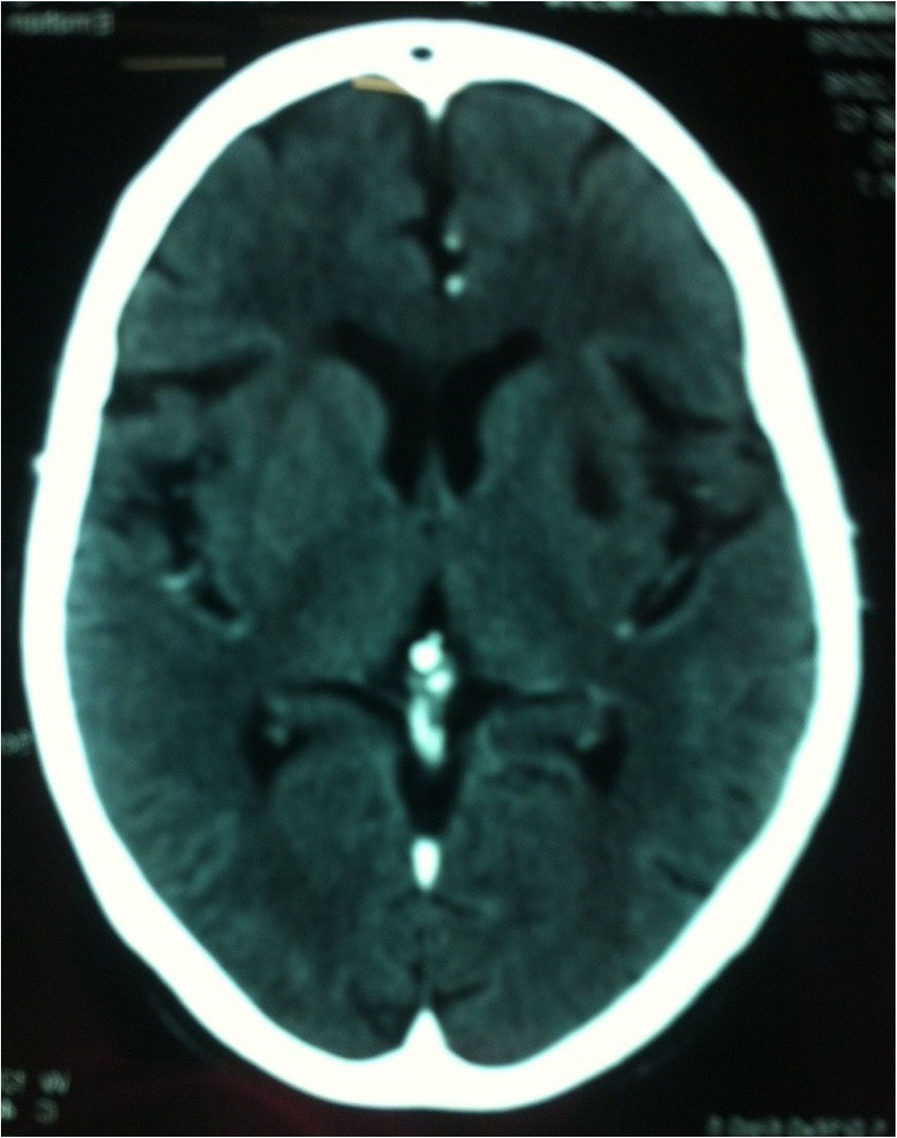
Non-contrast CT scan of the brain demonstrating an infarction in the region of the putamen on the left
